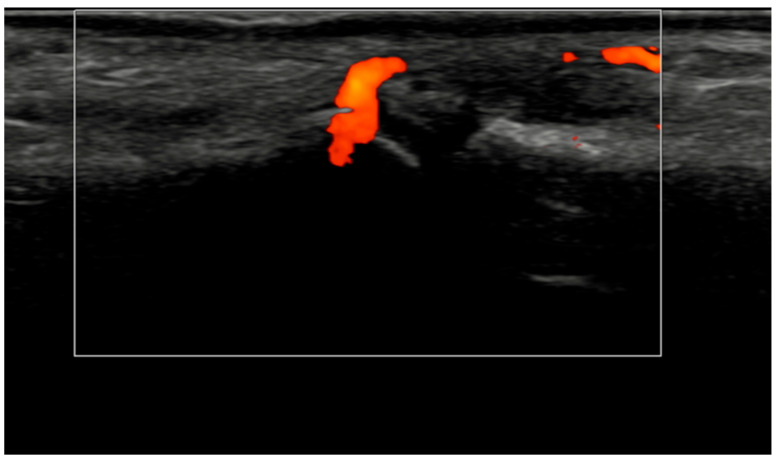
Longitudinal ultrasound image of the PIP III joint showing synovial hypertrophy (grade 2) and intra-articular power Doppler signal with distal capsular and entheseal extension (PD grade 2), corresponding to a combined EULAR–OMERACT synovitis grade = 2 (joint level).
